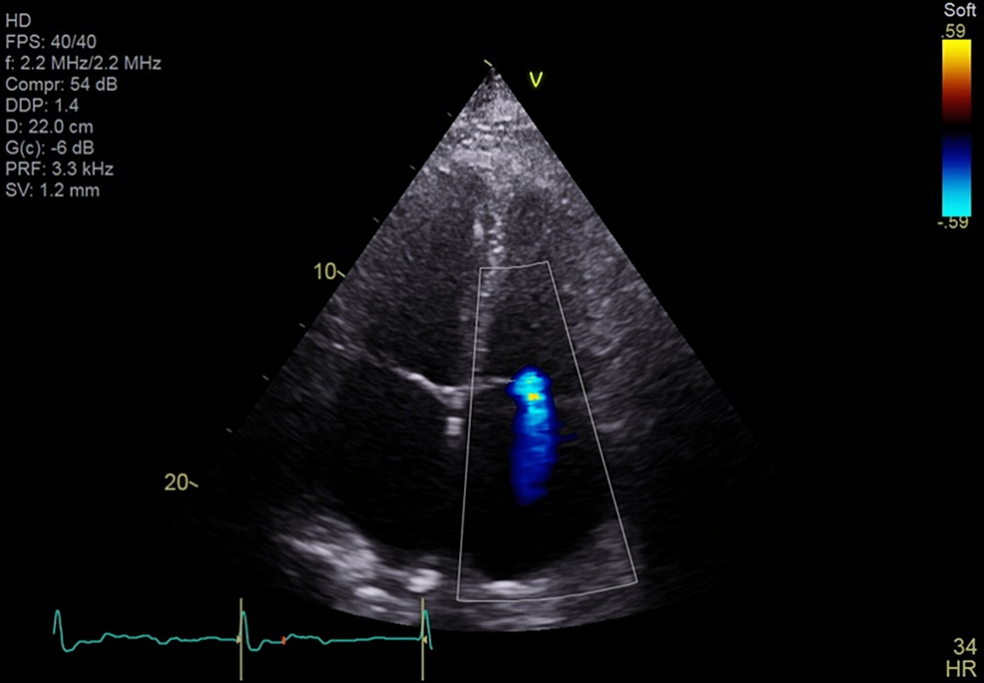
Baseline transthoracic echocardiogram of trivial tricuspid regurgitation prior to pacemaker placement. By Mayo Clinic convention, the left ventricle is inverted on the image (left upper cardiac chamber on image).
